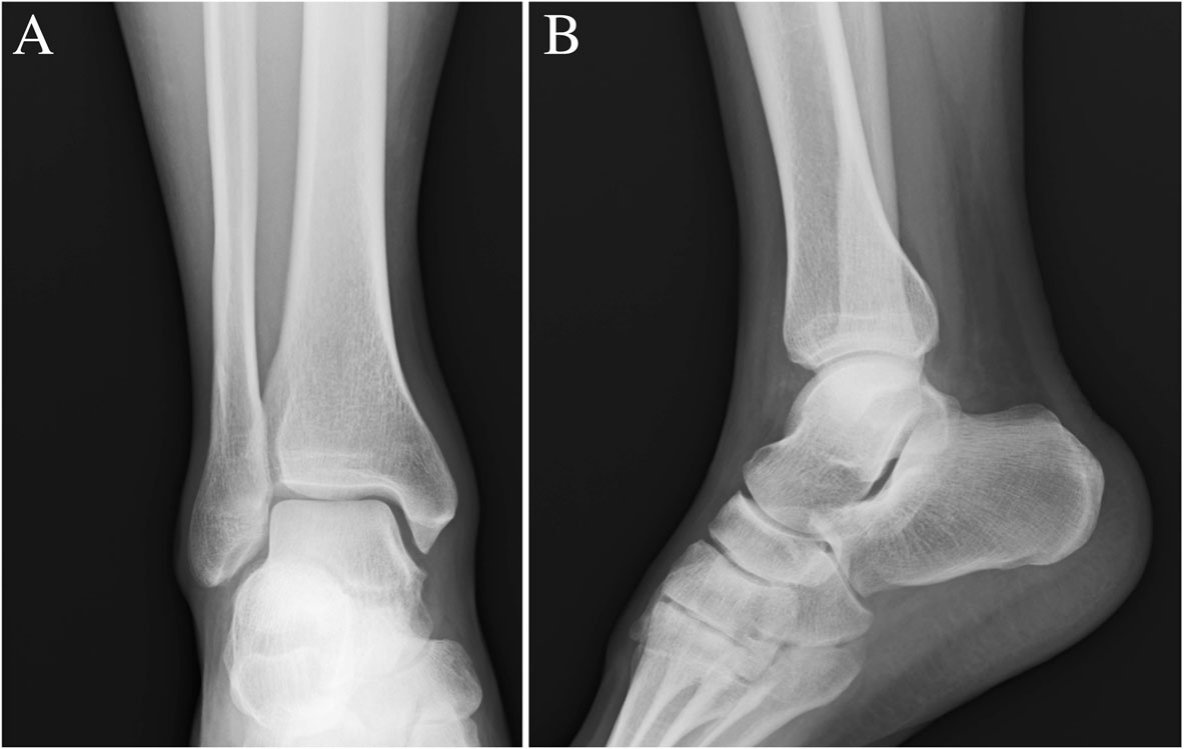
Standard radiographs of the ankle show a normal appearance. a Anteroposterior view. b Lateral view
